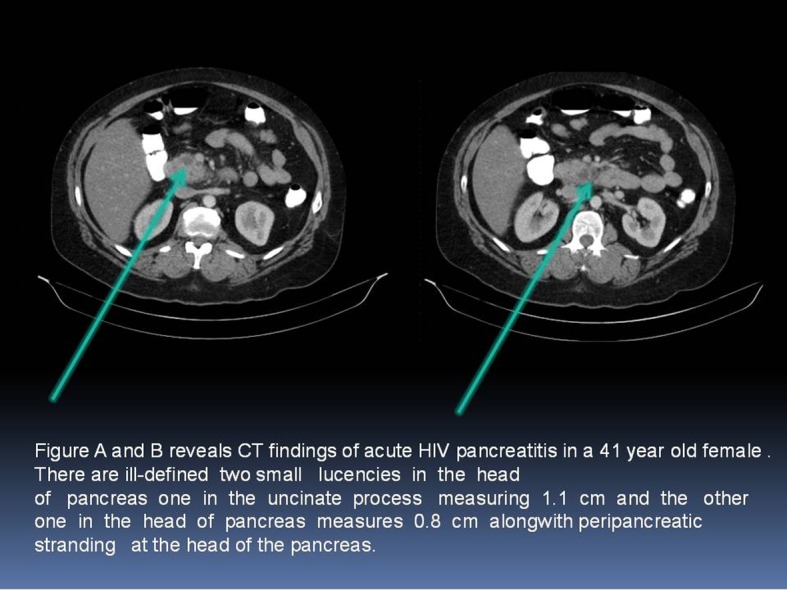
A and B: CT scan of acute pancreatitis in a 41-year-old female showing lucencies in the head of the pancreas and peripancreatic fluid stranding.
